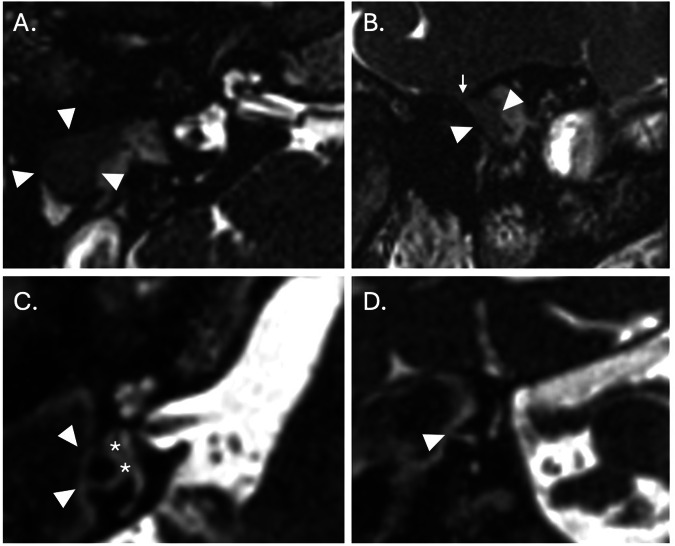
Cholesteatoma-mediated bone erosion on MRI. Axial (A) and sagittal (B) CISS MRI sections through the temporal bone of a patient who had previously undergone a CWUM for cholesteatoma. There is a defect in the tegmen mastoideum (small arrow) through which brain parenchyma (white arrowheads) herniates and demonstrates a lower signal than the surrounding middle ear fluid. Axial (C) and coronal (D) CISS MRI sections through the right temporal bone of a patient with an expanding cholesteatoma matrix causing erosion of the lateral semicircular canal (white arrowheads) and a reduction in perilymphatic signal locally (asterisks) in keeping with increased protein content
